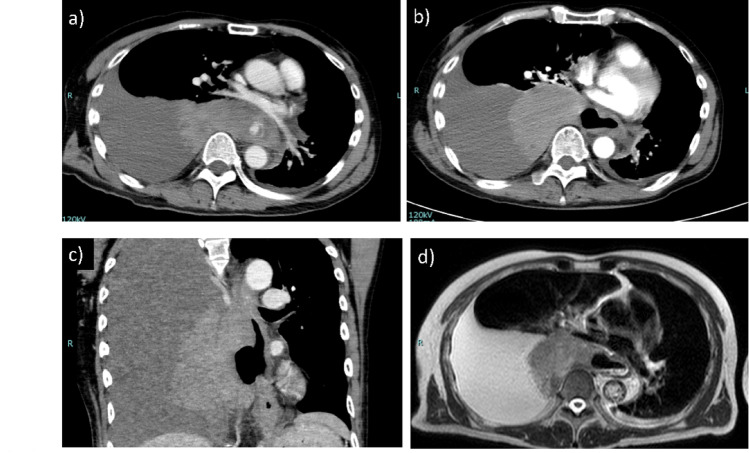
a Contrast-enhanced chest CT showing a mediastinal mass-like lesion along the mid-thoracic esophagus with right pleural effusion. b, c Contrast-enhanced chest CT demonstrating a diverticulum-like lesion in the right wall of the mid-thoracic esophagus. d T2-weighted MRI showing a homogeneous high-intensity lesion along the esophageal wall, consistent with an intramural hematoma
